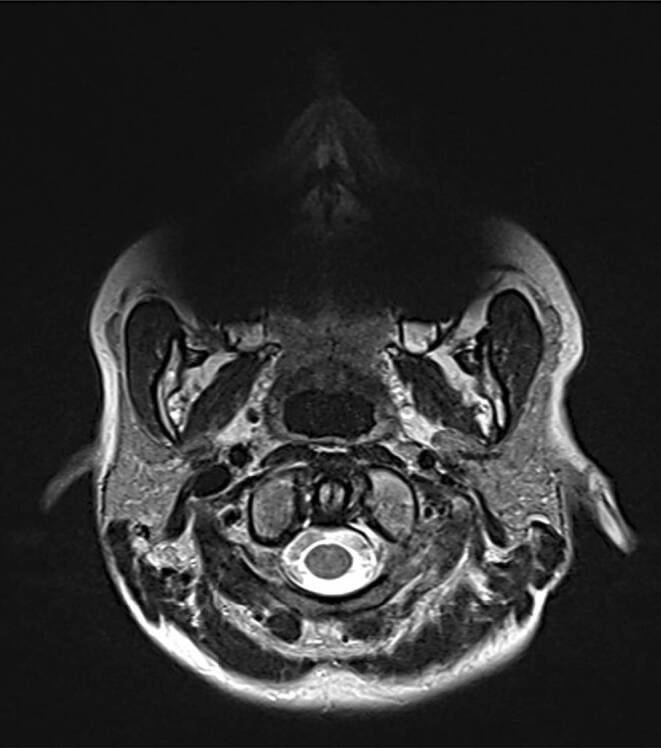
Patient A: MRI image (1.5 T): transversal section at the level of the *dens axis*, T2-weighted TSE sequence (TR: 5.700/TE: 112). Ceramic brackets on the anterior teeth, metal brackets on premolars and molars, made of the metastable austenitic steel grade 1.4301 (Dental-Vertrieb 2000 GmbH, Alsbach-Hähnlein, Germany). Measured permeability µ_r_ = 1.472. Limited assessability due to susceptibility artifacts Patient A: MRT-Bild (1,5 T): transversaler Schnitt auf Höhe des *Dens axis*, T2-gewichtete TSE-Sequenz (TR: 5.700/TE: 112). Keramikbrackets an den Frontzähnen, die Metallbrackets an den Prämolaren und Molaren sind aus dem metastabile austenitischen Stahl 1.4301 (Dental-Vertrieb 2000 GmbH, Alsbach-Hähnlein/Deutschland) hergestellt. Gemessene Permeabilität µ_r_ = 1,472. Eingeschränkte Beurteilbarkeit aufgrund von Suszeptibilitätsartefakten
